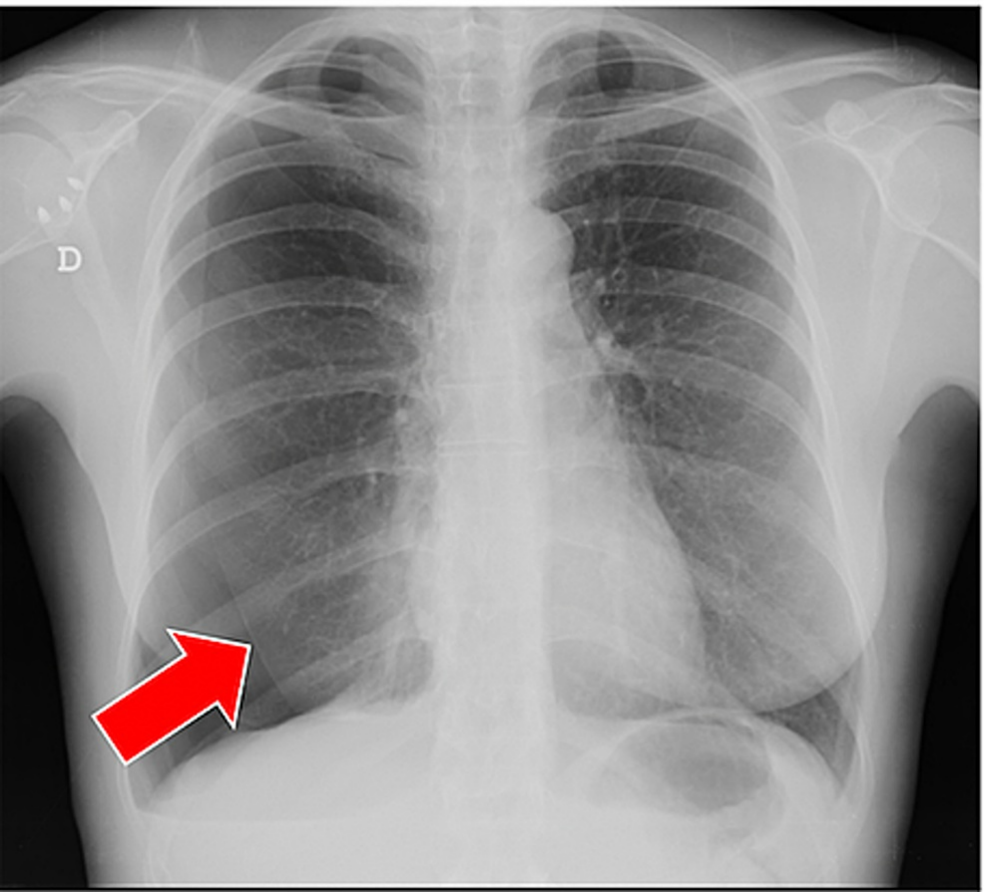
Upright posterior–anterior chest radiograph Upright posterior–anterior chest radiograph revealing a right spontaneous pneumothorax during menses (*katamênios* in greek). The subtle extrapulmonary increased lucency of the pneumothorax is outlined by the visceral pleura, a sharp thin radiopaque linear shadow (red arrow). The lung is partially collapsed with no lung markings beyond the visceral pleura.
